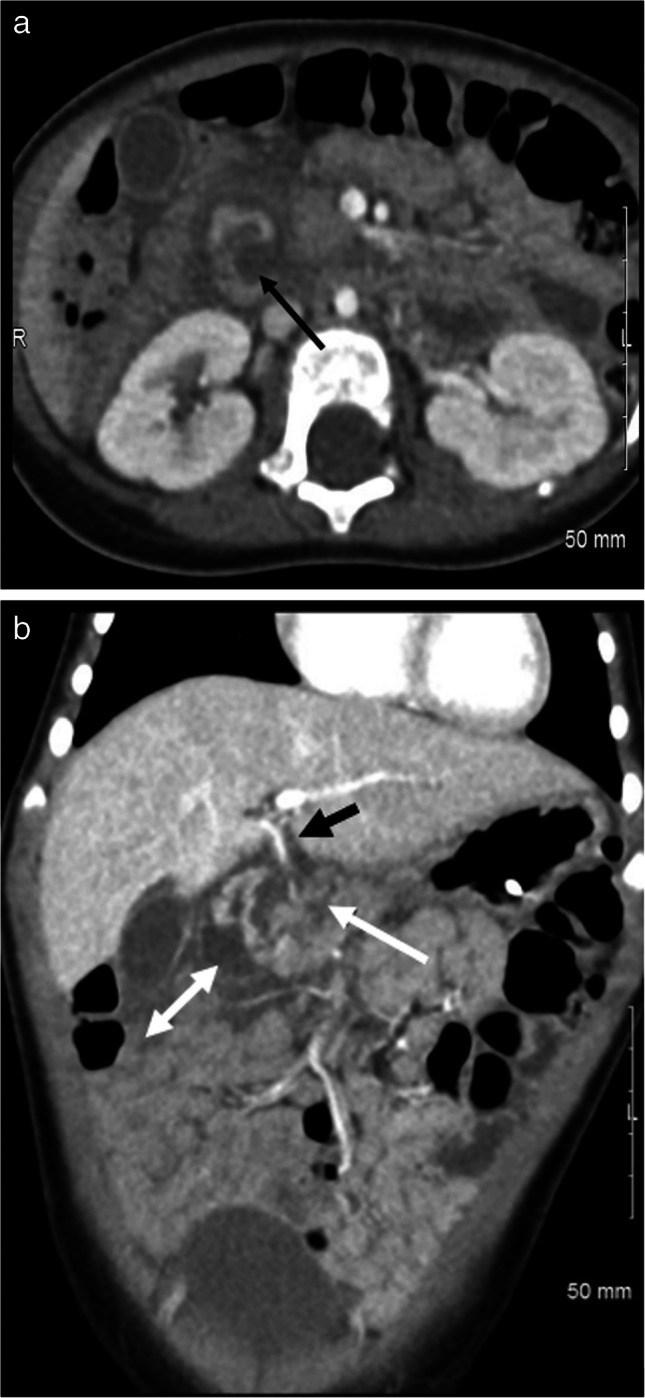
A 13-month-old male with no reported trauma history who presented with bilious emesis and underwent a CT of the abdomen and pelvis with IV contrast and no oral contrast in portal venous phase. Findings elicited concern for physical abuse. a Axial image shows a focal intramural lesion compatible with a duodenal hematoma (*black arrow*) in the medial wall of the second portion of the duodenum, near the ampulla of Vater. b Coronal image of the same study shows a dilated common duct (*black arrow*) and pancreatic duct (*white arrow*) due to obstruction of the ampulla of Vater by the duodenal hematoma (*double-headed white arrow*)
